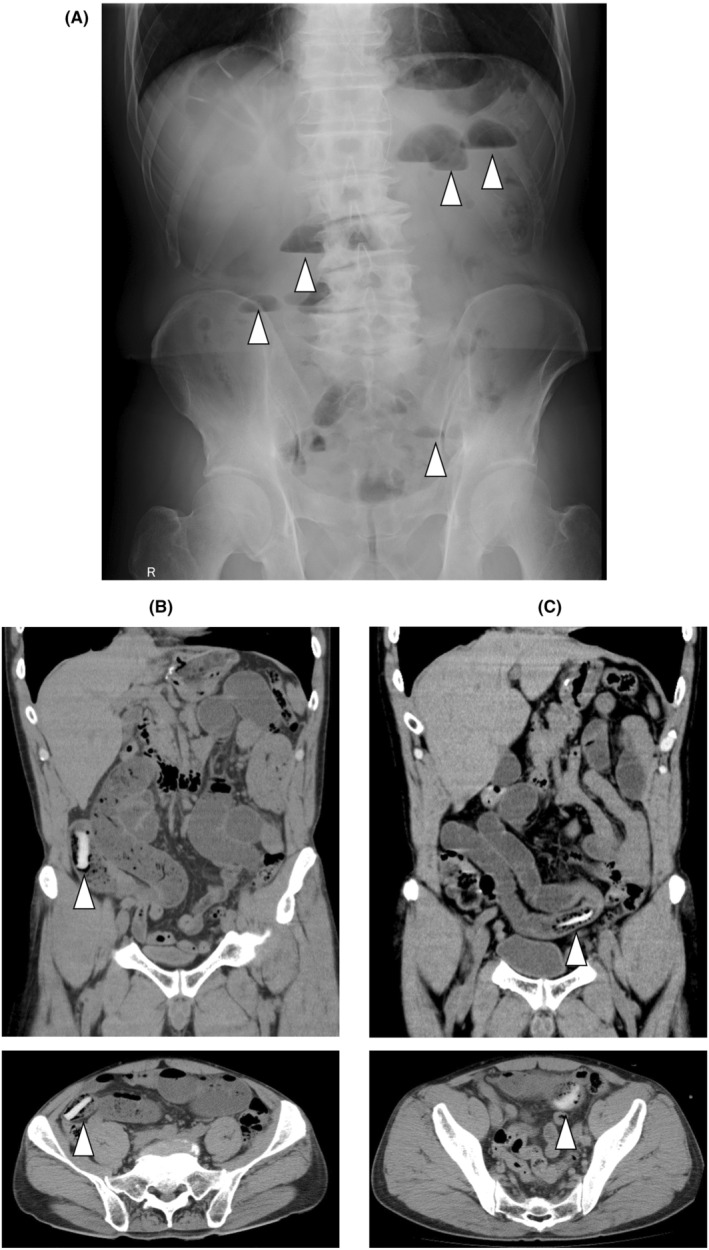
(A) The patient's abdominal X‐ray demonstrated a stepladder appearance with sentinel air retention, which suggested alimentary tract obstruction. (B) The patient's abdominal computed tomography (CT) scans revealed a 4‐cm‐long flat‐type white matter adjacent to his jejunum, which indicated intestinal obstruction due to a high‐density food. (C) A day after admission, the patient's abdominal CT scans showed resolution of intestinal obstruction with a melted high‐density object moved to the anal side.
